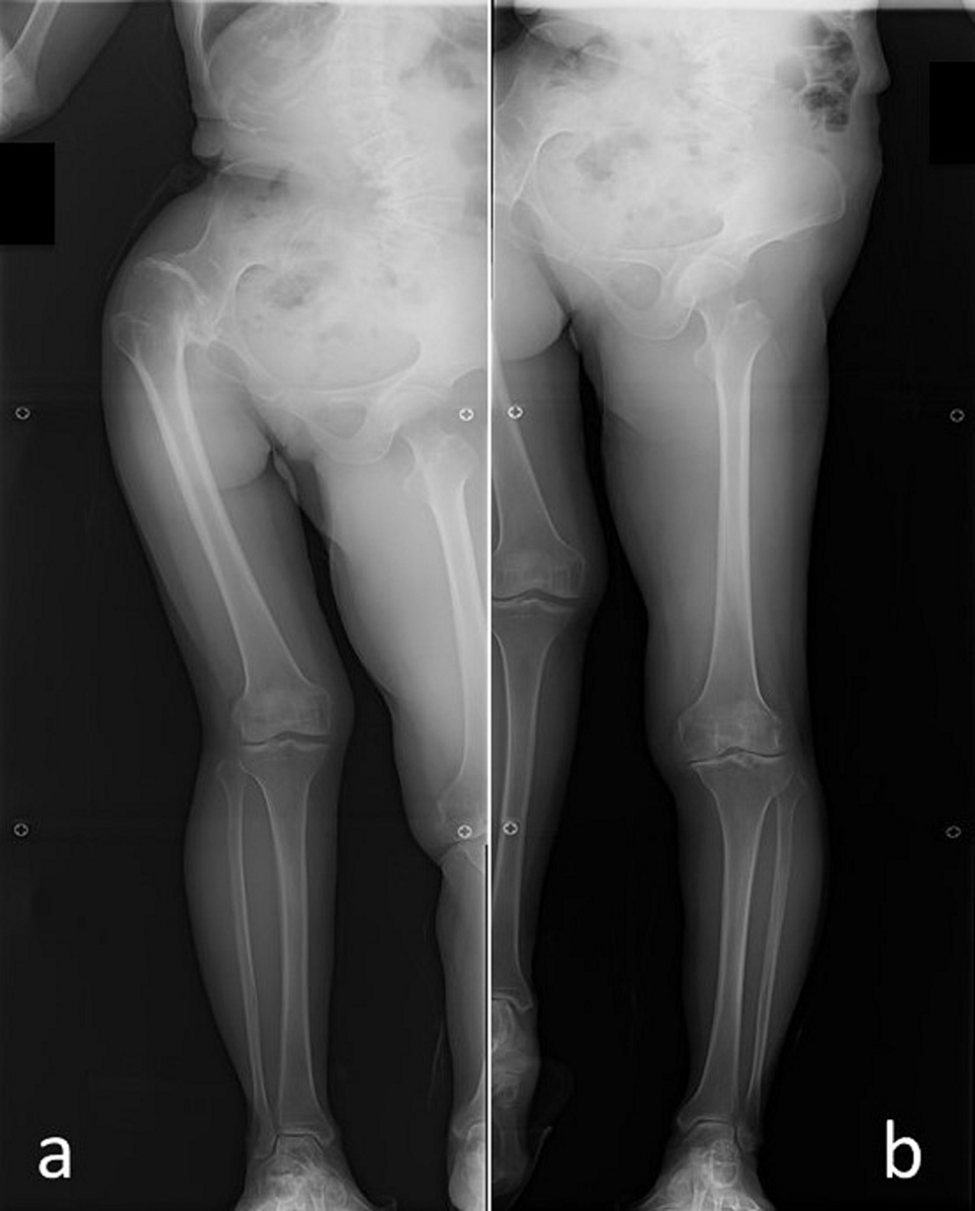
Antero-posterior radiographs of the lower extremities in standing postures. Natural standing posture represents excessive knee flexion of the longer (left) leg to adjust for the LLD (a). On the complete extension of the left knee, she was forced to stand on the toes of her affected right foot, causing plantar flexion of the foot of the shorter limb (b).
